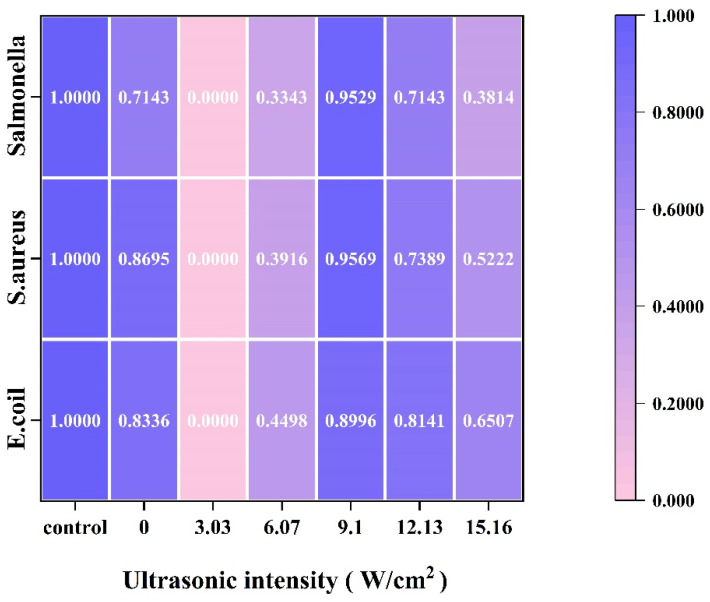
Effect of ultrasonic intensity on the bacteriostatic activity of CPP proteins during UA acid extraction. Control was a commercially available standard.
